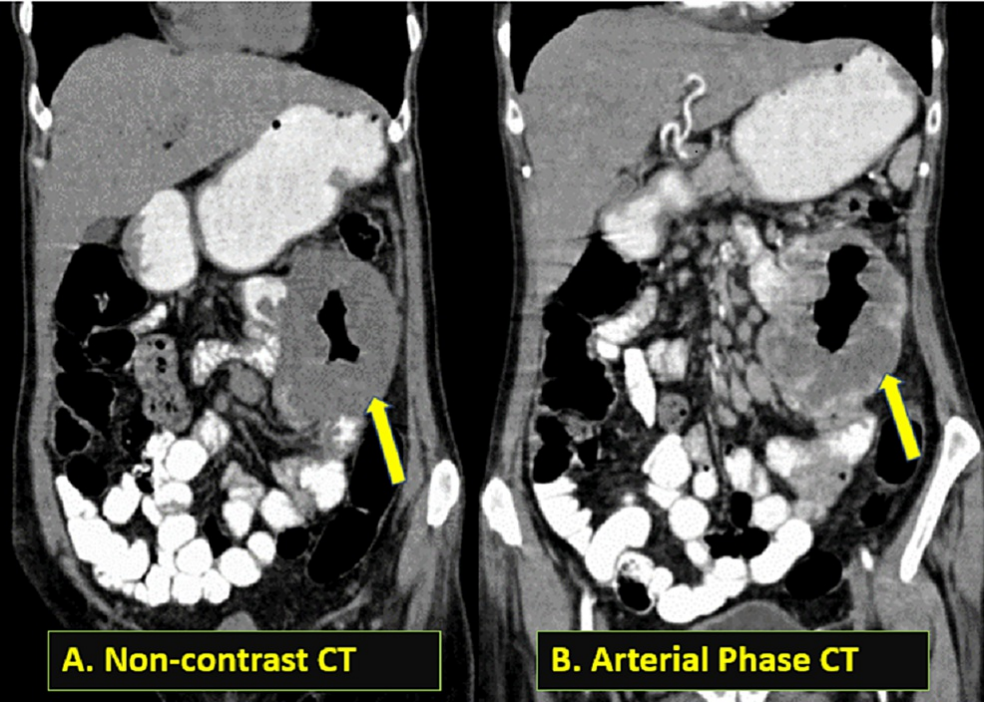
(A) Coronal section, non-contrast computed tomography image (soft tissue window) showing a heterogeneous mass lesion (shown by the arrow) with central necrosis in the left hypochondrium abutting the jejunum with no signs of intestinal obstruction. (B) Coronal section, arterial phase-contrast enhanced computed tomography image (soft tissue window) of a mass lesion with peripheral enhancement (shown by the arrow) and multiple enlarged enhancing pre-aortic, para-aortic, and mesenteric lymph nodes.
